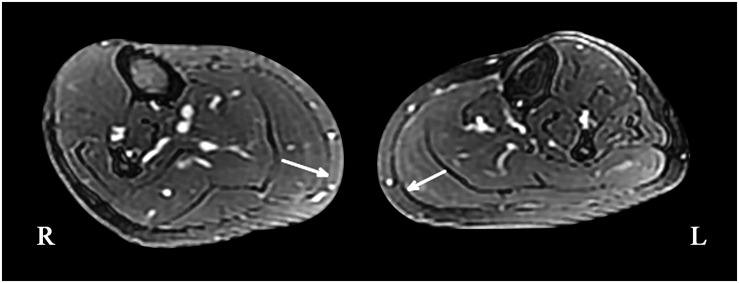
Magnetic resonance imaging of the bilateral calf muscles; asymmetry of the posterior muscles of the left calf can be seen on cross-section (maximum diameter: right side, 21.53 mm; left side, 17.03 mm).
